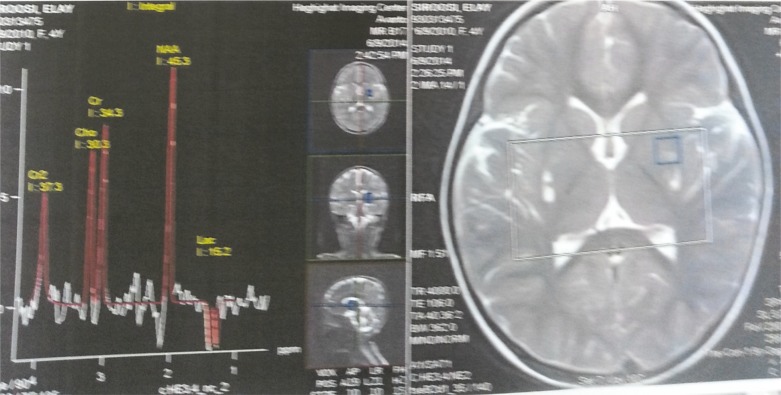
Cranial MRI and MRS in a patient with glutaric aciduria type I and Axial MRI shows an abnormal signal in bilateral basal ganglia with bilateral prominent sylvian fissures
